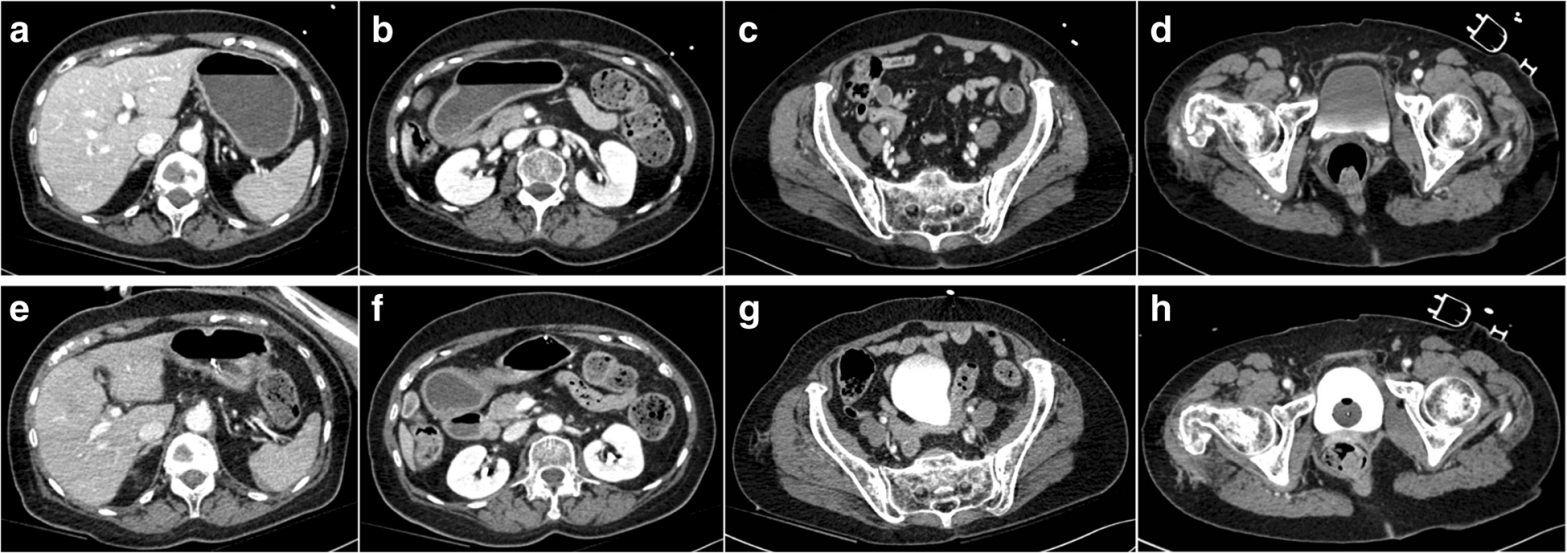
Upper series* (a-d): First trauma computed tomography scan without any signs for free abdominal fluid or air, no signs for the extravasation of the radiocontrast agent in the abdominal or pelvic cavity (indicating a vascular injury), and no signs for a greater hematoma in the small pelvis. *Lower series* (e-h): Second trauma computed tomography scan 90 minutes later still revealed no free abdominal fluid or air, no signs of extravasation of the radiocontrast agent in the thoracic, abdominal, or pelvic cavity, and no signs for a progressing hematoma of the spleen or in the small pelvis
